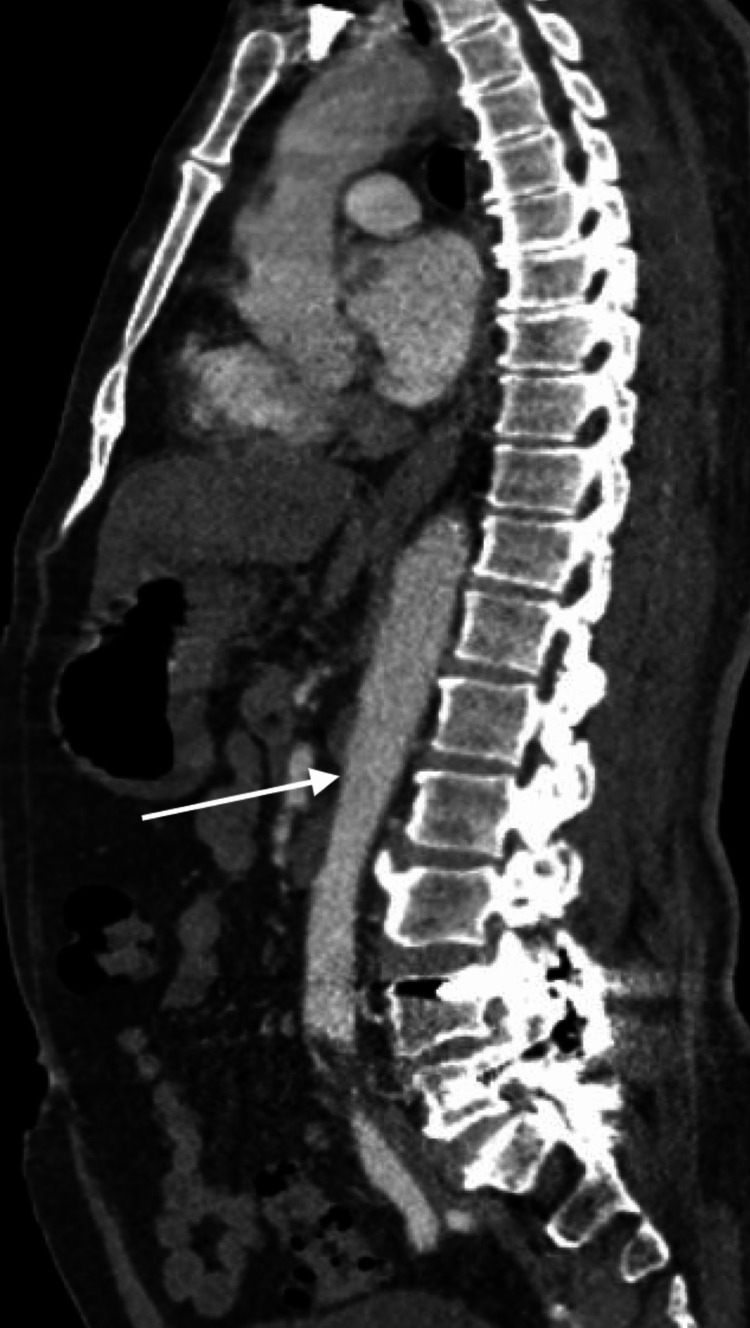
Computed tomography angiography of the chest, abdomen, and pelvis demonstrating normal thoracic and abdominal aorta
